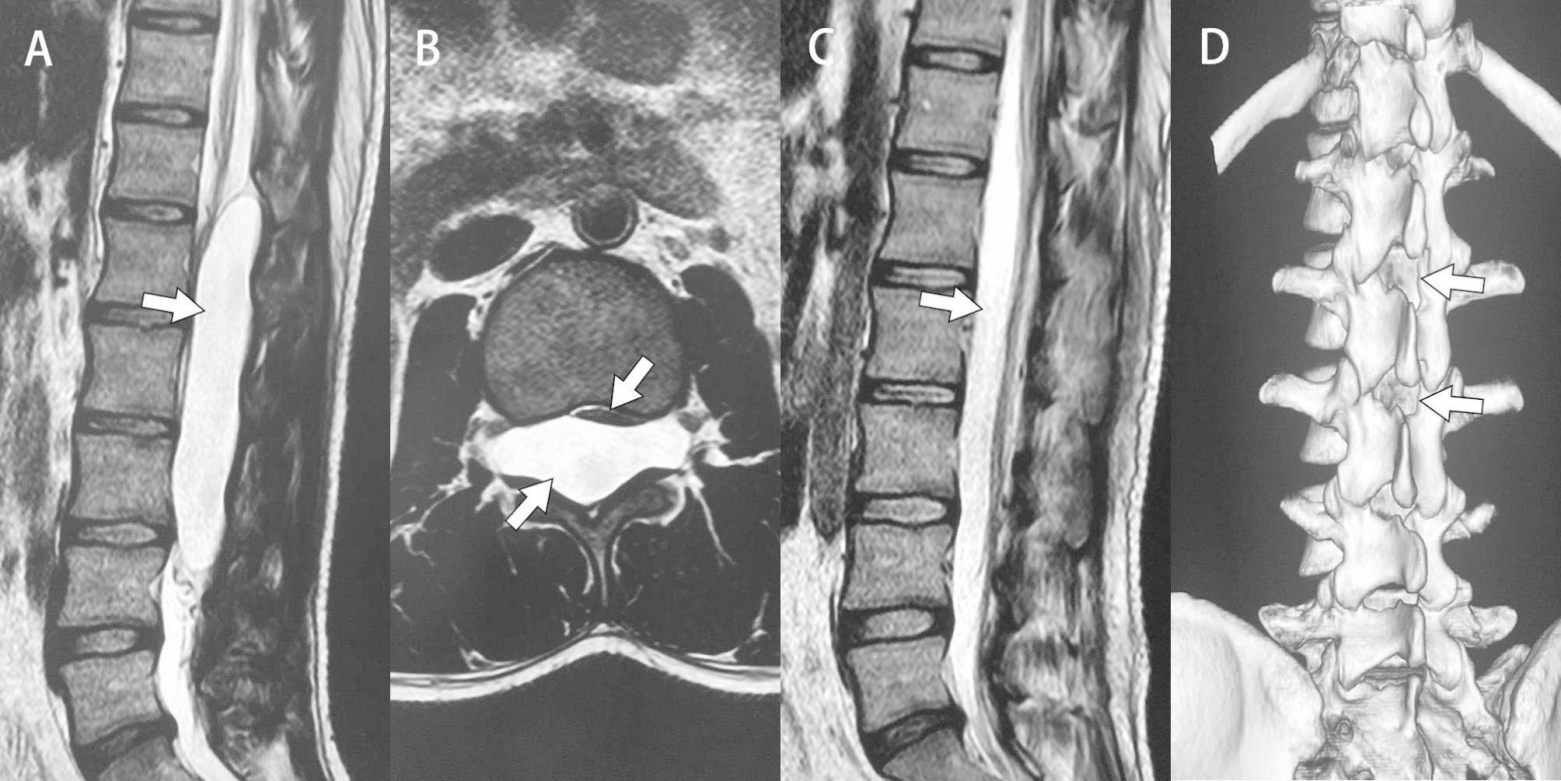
Patient 3 MR imaging data. A: Preoperative sagittal T2WI image. (Arrow: A large SEAC from lower T12 to upper L4.) B: Preoperative axial T2WI image. (Upper arrow: The compressed dural sac. Lower arrow: The SEAC was located dorsally within the spinal canal and severely compressed the cord.) C: Postoperative sagittal T2WI image. (Arrow: The cyst has been completely removed and the space of the spinal canal was also restored.) D: 3D reconstructed image of the spine. (Upper arrow: The position of the lamina fenestration, we closed the fistula orifice and removed the upper part of the cyst wall. Lower arrow: The position of the lamina fenestration, we removed the lower part of the cyst wall.)
